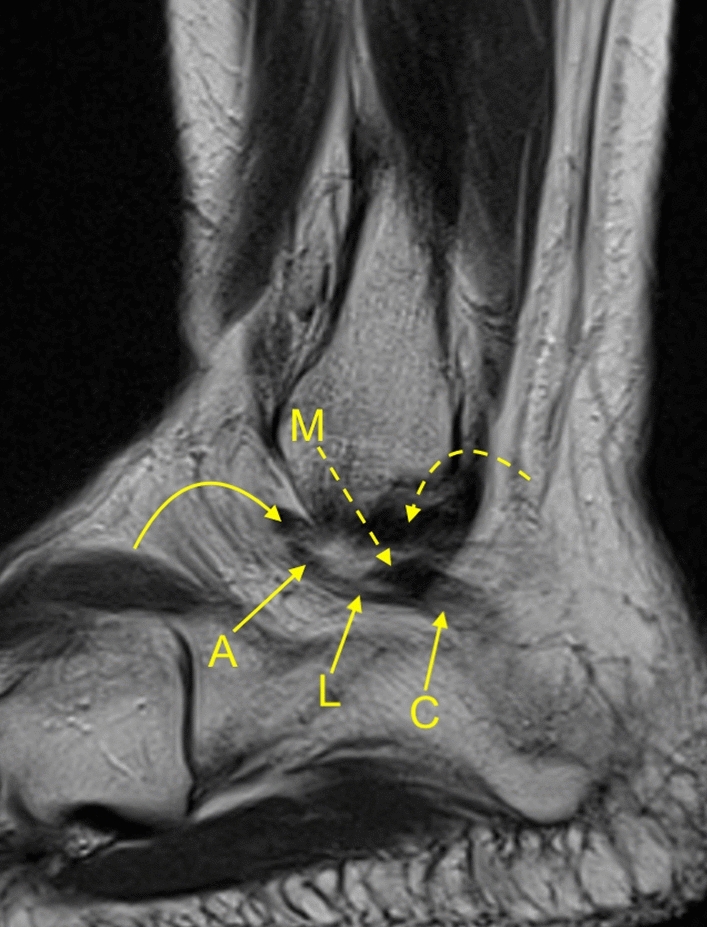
A 37-yeard-old patient with suspicion of a stress fracture of the navicular bone, which was not confirmed on the MRI. The arch-shaped communication between the ATFL (A), the L-CFL (L), the M-CFL (M), and the PTFL (curved dashed arrow) on the sagittal section.
